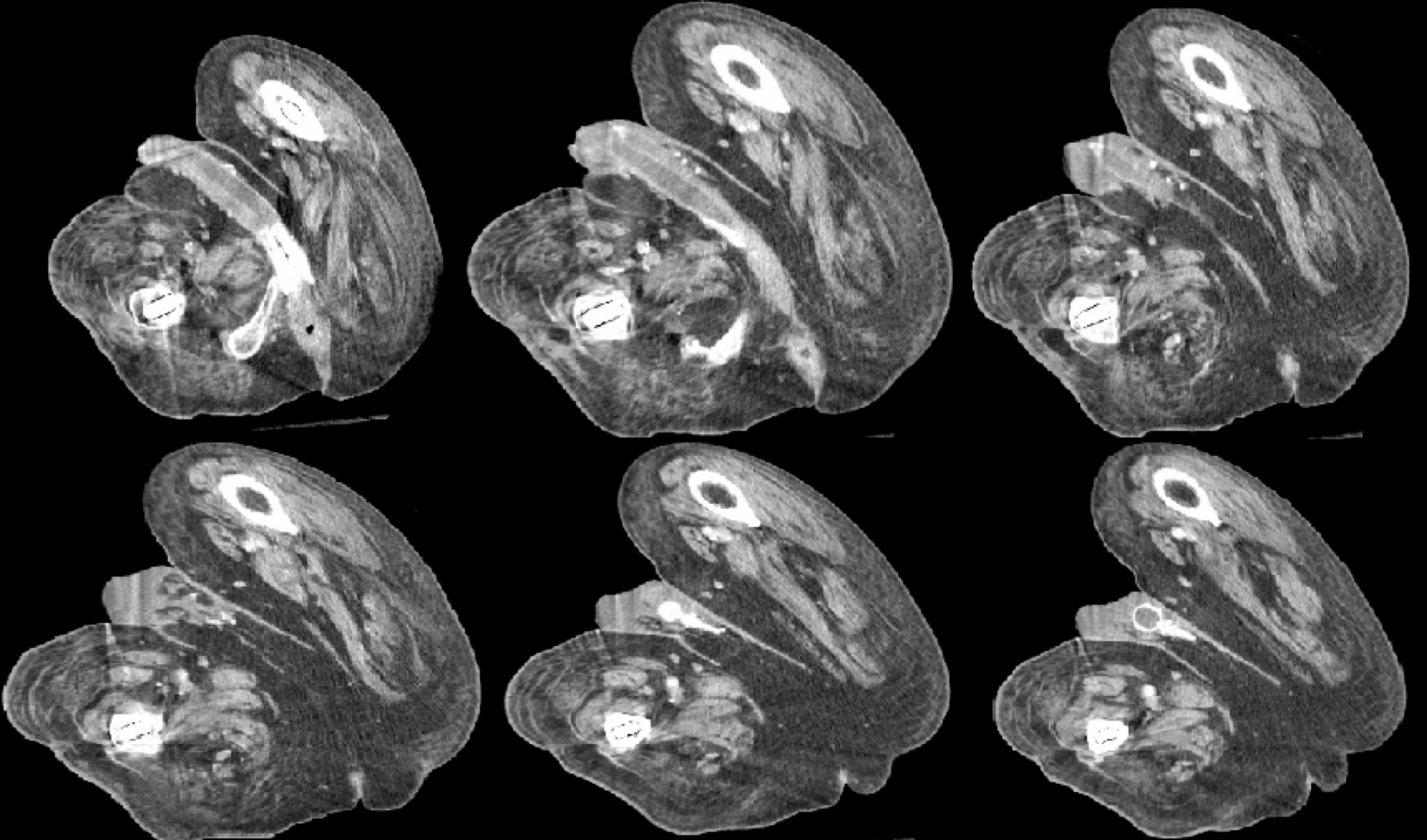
Serial contrast-enhanced CT scan (axial) images of the pelvis, progressing craniocaudally Bilateral intracavernosal penile prosthesis cylinders are seen with a small surrounding low-attenuation fluid collection and adjacent mild soft-tissue stranding, with beam-hardening artifact related to bilateral femoral implants.
